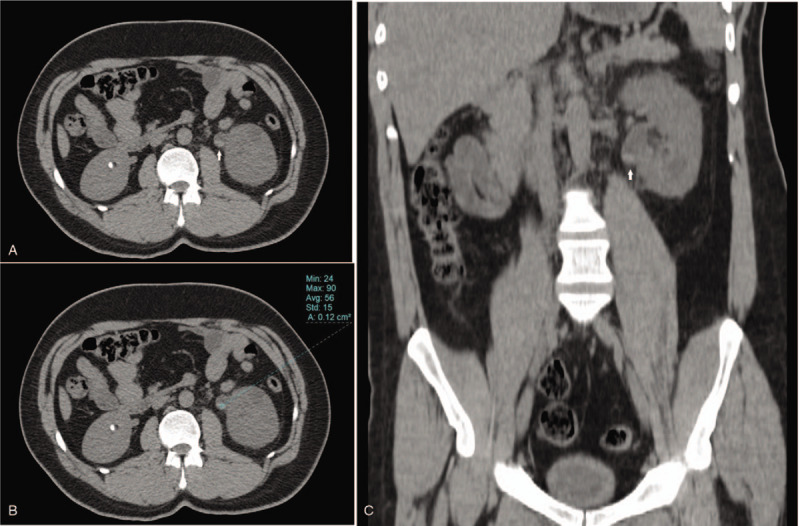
Axial (A, B) and coronal reformatted (C) unenhanced computed tomography (CT) images demonstrate hyperdense lesion in the left ureteropelvic junction (arrows). There is also small stone in the right kidney.
